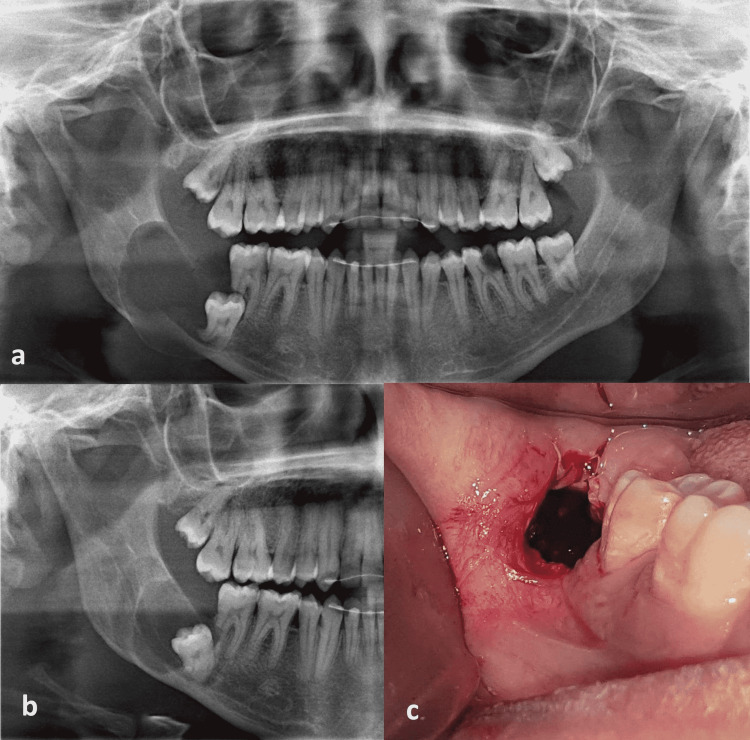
Initial panoramic radiograph (a) A large cystic lesion and the impacted 3rd molar (b) Six months after needle aspiration, the cystic cavity shrank (c) A window was created as a part of marsupialization.
